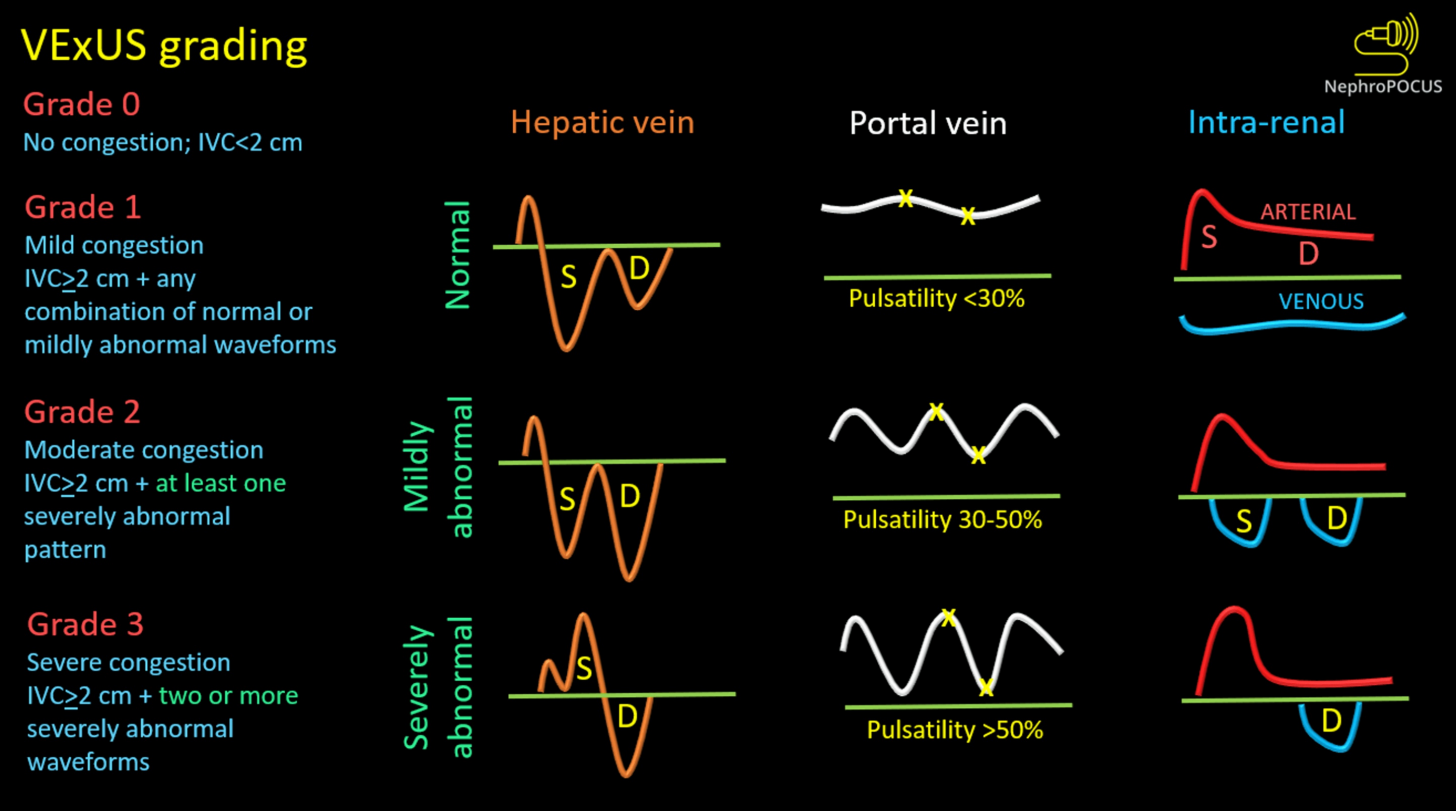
VExUS grading When the diameter of the IVC is >2 cm, three grades of congestion are defined based on the severity of abnormalities on the hepatic, portal, and renal parenchymal venous Doppler. Hepatic vein Doppler is considered mildly abnormal when the systolic (S) wave is smaller than the diastolic (D) wave but still below the baseline; it is considered severely abnormal when the S-wave is reversed. Portal vein Doppler is considered mildly abnormal when the pulsatility is 30-50%, and severely abnormal when it is ≥50%. Asterisks represent points of pulsatility measurement. Renal parenchymal vein Doppler is mildly abnormal when it is pulsatile with distinct S and D components and severely abnormal when it is monophasic with a D-only pattern. IVC: inferior vena cava; VExUS: venous excess ultrasound Adapted from NephroPOCUS.com with permission
